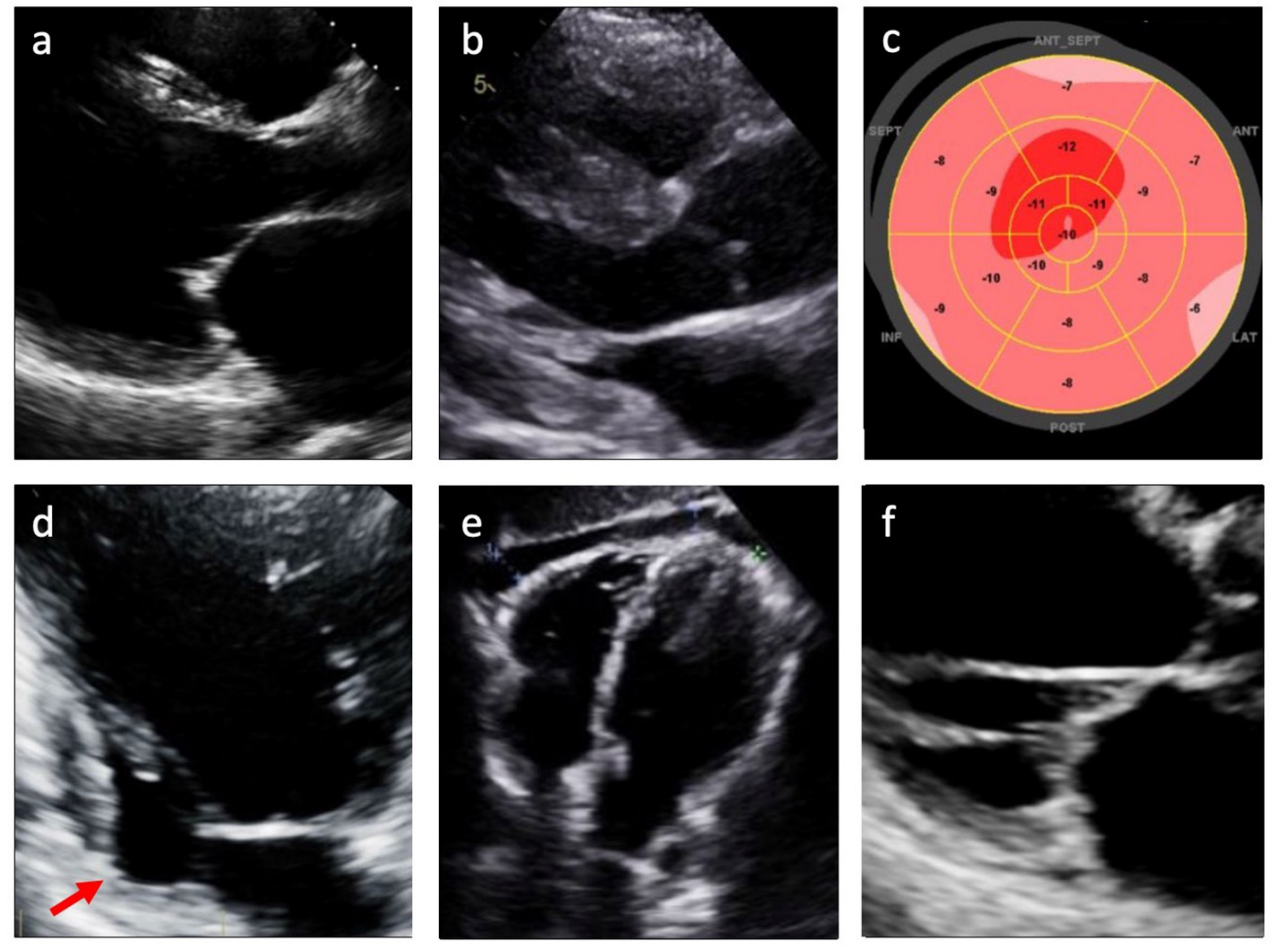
Echocardiographic findings in cardiac sarcoidosis. (a) Left ventricular dilatation; (b) Left and/or right ventricular hypertrophy; (c) Reduced global longitudinal strain (GLS); (d) Left ventricular wall aneurysm (arrow); (e) Pericardial effusion; (f) Valvular thickening or dysfunction.
